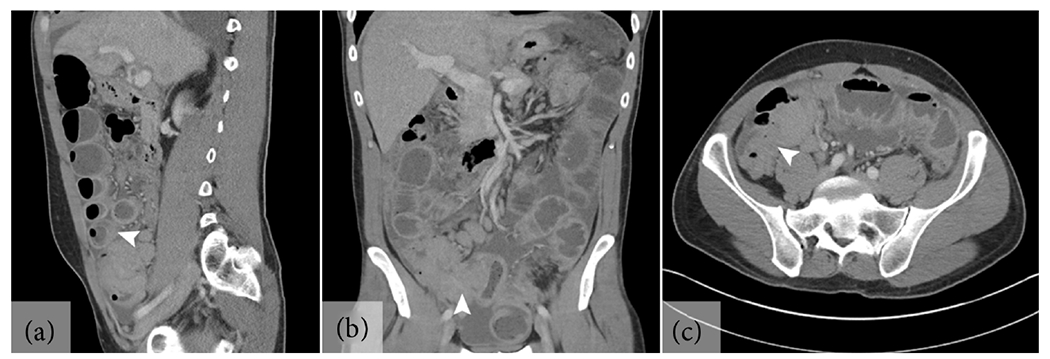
Computed tomography imaging of early ileocolic intussusception. (a) Sagittal view showing dilated bowel with air fluid levels and an early distal target sign (arrowhead). (b) Coronal view showing the transition point centered on an ileocecal mass (arrowhead). (c) Axial view of the ileocecal mass (arrowhead) and air-fluid levels.
